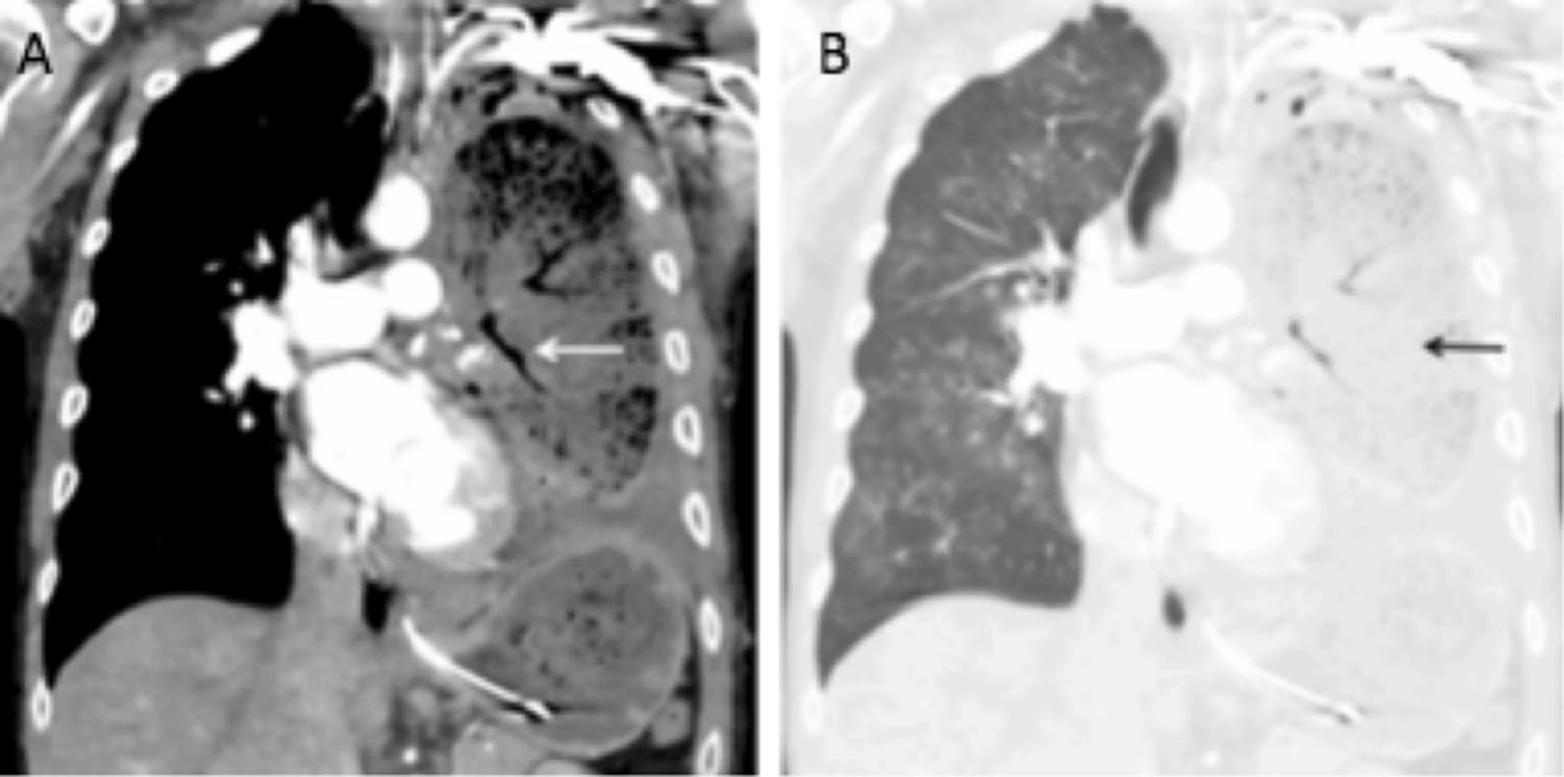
Lobar torsion. Coronal CT images (A: mediastinal window, B parenchymal window) showing a left lower lobar torsion after left upper lobectomy. A CT shows the torsion of the pedicle (white arrow) and the ascent of the left lower lobe. B We notice the condensation of the remaining lobe (black arrow)J.
